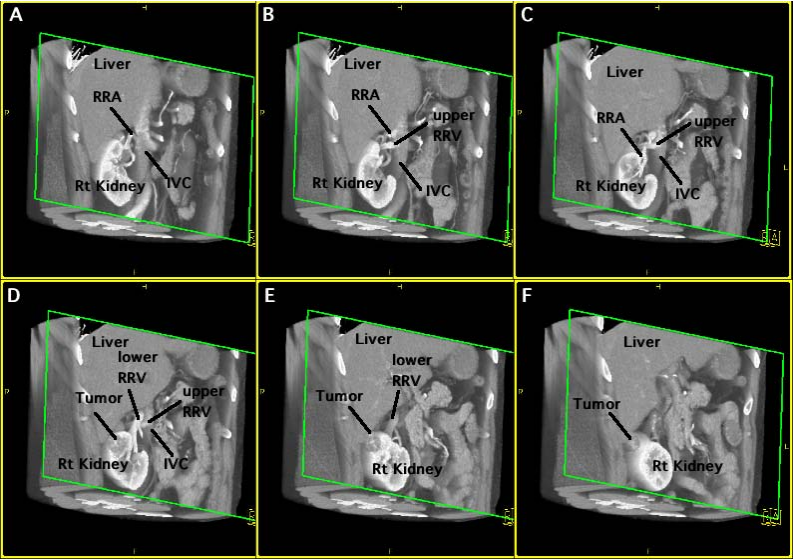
A right renal tumor and two renal veins. Oblique view from the ventral to dorsal direction in the arterial phase. The CT scans are arranged from dorsal (A) to ventral (F). A: The right renal artery (RRA) is seen to the right of the inferior vena cava (IVC). B: The upper right renal vein (RRV) can be seen. C: The anterior branches of the RRA and RRV cross each other. D: The lower RRV drains into the IVC closer to the heart than the upper RRV, indicating that these both veins cross over each other. E, F: The tumor is located in the upper pole of the kidney.
